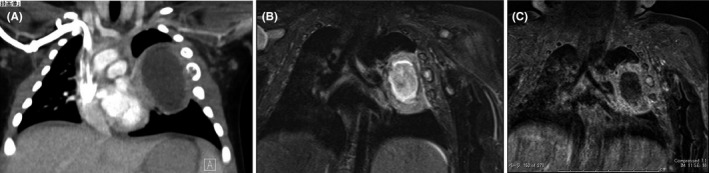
Computed tomography showing a lung abscess (4 × 5 × 3 cm). The fourth rib adjacent to the mass was irregularly enlarged, and the cortex had been destroyed (A). Fat‐suppressed T2‐weighted (B) and postcontrast fat‐suppressed T1‐weighted (C) magnetic resonance imaging showing hypersignal intensity in the irregularly enlarged fourth rib, suggesting osteomyelitis. No abnormal signals were seen in the third or fifth ribs
